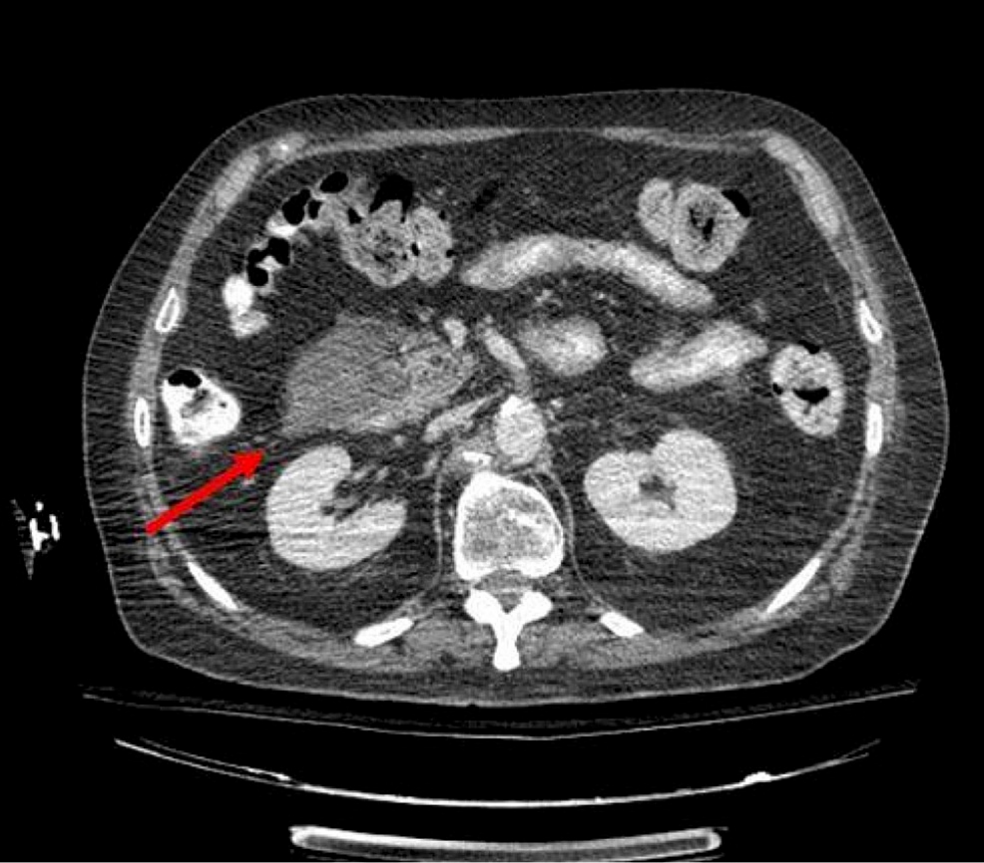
CT of abdomen and pelvis with intravenous contrast depicting a pancreatic head pseudocyst measuring 5.7x3.7 cm (arrow)
